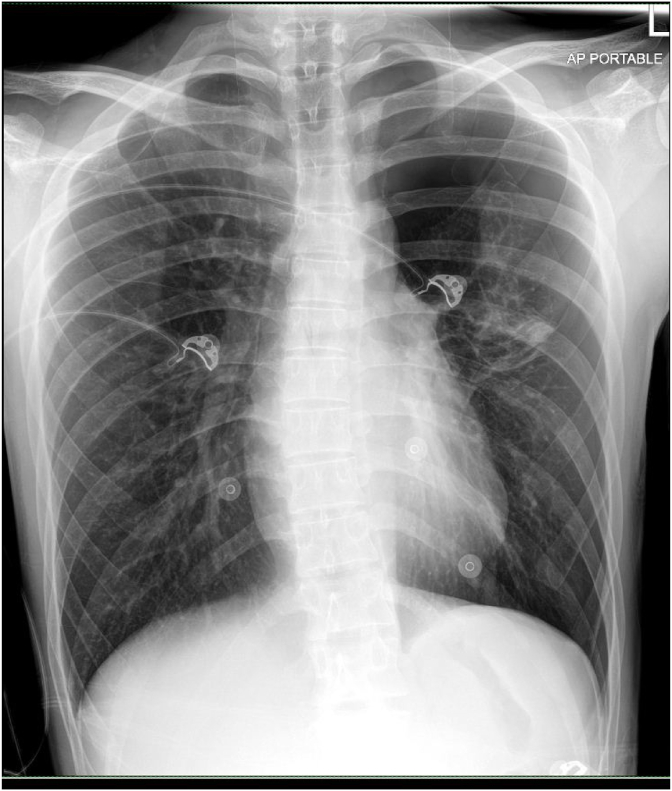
Plain chest radiograph erect showing left sided pneumothorax.
